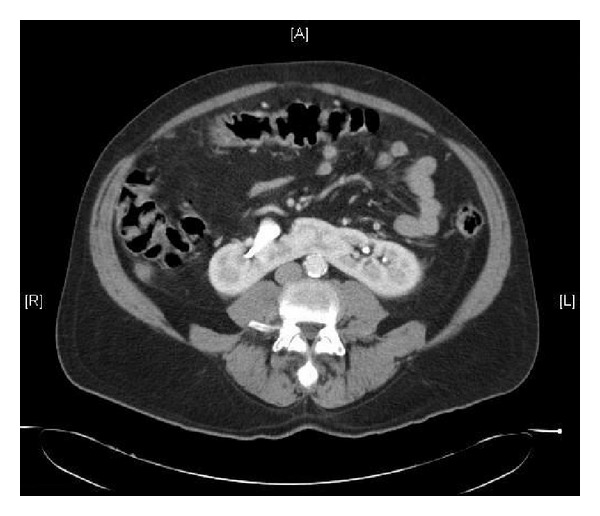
Donor CT scan. This image from the prerecovery donor CT scan shows the bridge of the horseshoe kidney and the inferior most portion of the collecting system noted on CT scan.
